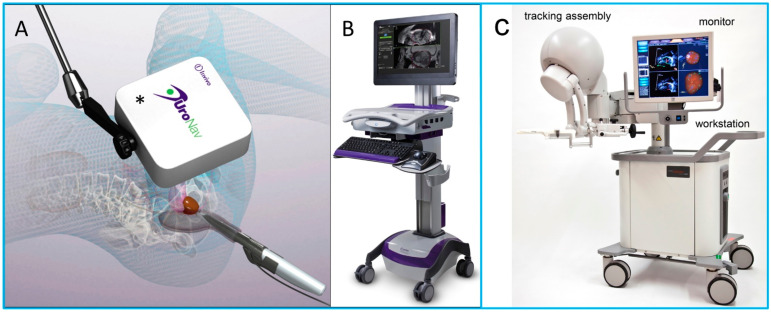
(A,B) Photo images of the UroNav US/MRI fusion system. (A) An electromagnetic field generator enables tracking of the transrectal US probe; (B) US/MRI fusion device. (C) Artemis US/MRI fusion system. Reprinted from [144] with permission.
